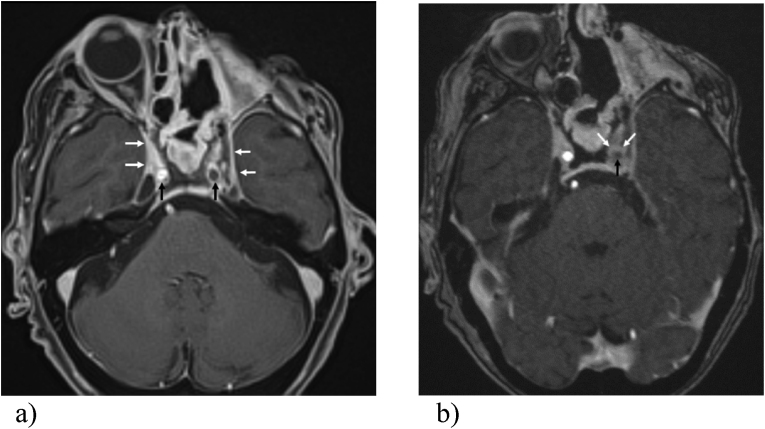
Follow-up MRI after left orbital exenteration. The axial T1-weighted starVIBE with fat saturation (slice thickness 1.5mm) after administration of intravenous contrast (Gadovist®) revealed progression of the inflammation on day 19 compared to day 3 and 6 with progressive bilateral involvement of the cavernous sinus (white arrows). A new filling defect of the left internal carotid artery is noted (black arrows), indicative of an occlusion of the vessel. The whole-brain post-contrast T1-weighted multiplane sequences showed perineural spread of the inflammation with extension along the course of the left mandibular nerve as well as contrast-enhancement of the right frontobasal pachymeninx. Fig. 2b)The 3D time-of -light MR angiography (slice thickness 0.6mm) after contrast confirmed the new occlusion of the left internal carotid artery with pathologically avid contrast enhancement of the thickened arterial wall (white arrows) and the missing flow void (black arrow). These MR images were performed on 3-T scanners (Siemens, Erlangen, Germany) 6 weeks after the initial diagnosis of the mucormycosis.
